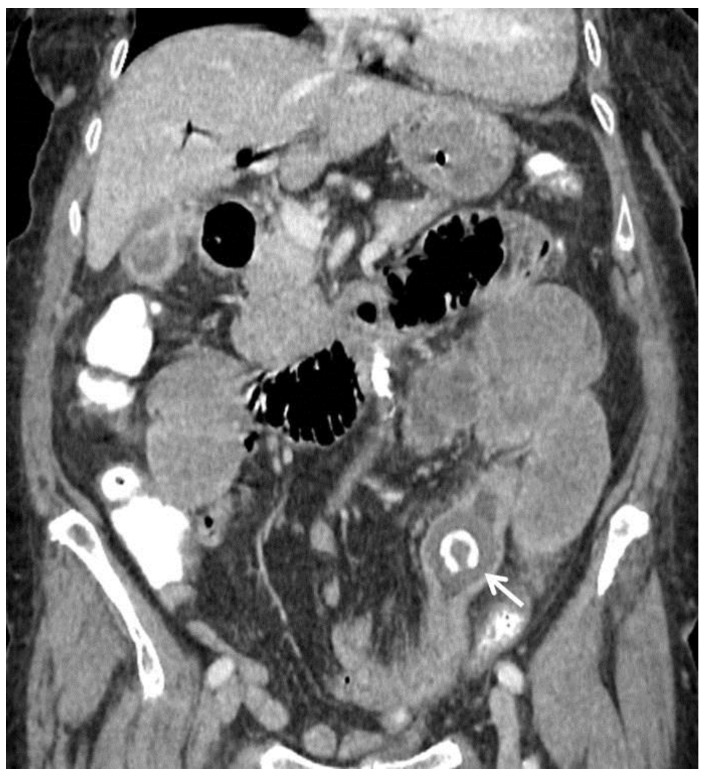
82-year-old female. Abdominal CT scan, coronal plane, portovenous phase. The transition point of the obstruction is visible, the gall stone is obstructing the small bowel lumen (arrow), distal loops are collapsed.
